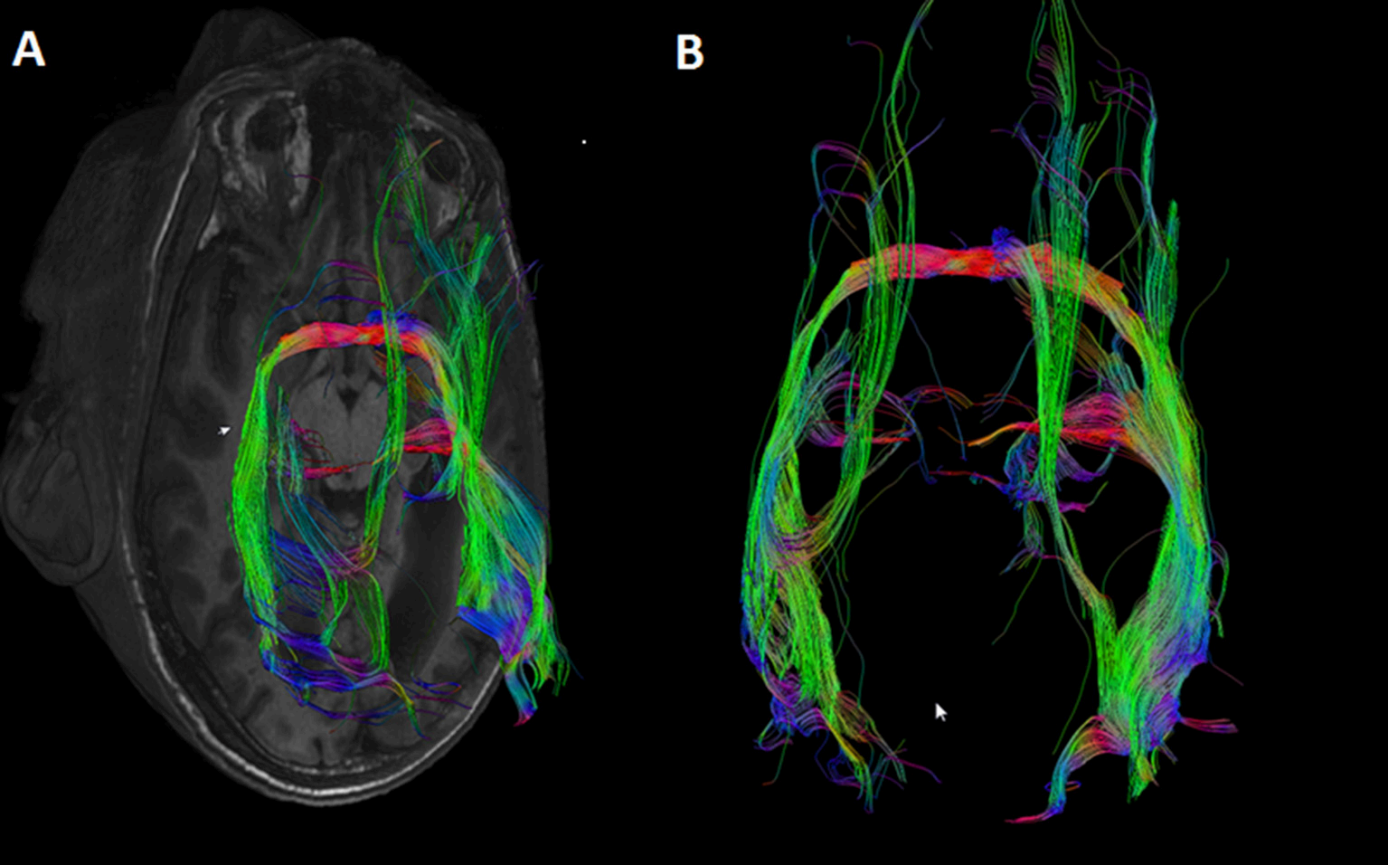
DTI tracts going through the anterior commissure in the individual in which the corpus callosum was missing. Seeds were placed in the anterior commissure without indicating any other region of interest. Large tracts project to the occipital cortical tips on both sides. (A) Axial MRI section with superimposed DTI tracts. (B) DTI tracts from superior (anterior side of the head on top of the image).
